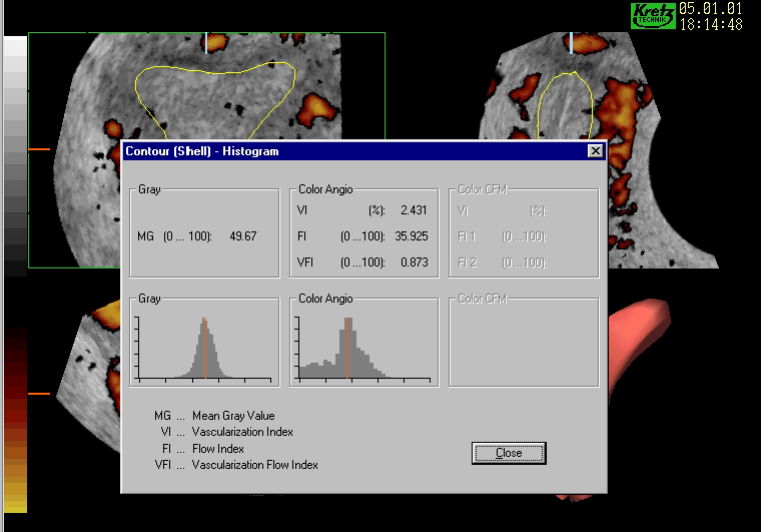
The 'Histogram'*. The three indices of vascularity have been calculated for the endometrial model together with the mean grey value. The Vascularisation Index (VI) reflects the degree of power Doppler information within the model and is considered as a percentage therefore whilst the Flow Index (FI) and Vascularisation Flow Index (VFI) include information on the mean power Doppler signal intensity referenced against a scale from zero to one hundred to indicate the minimal and maximum range accordingly.
